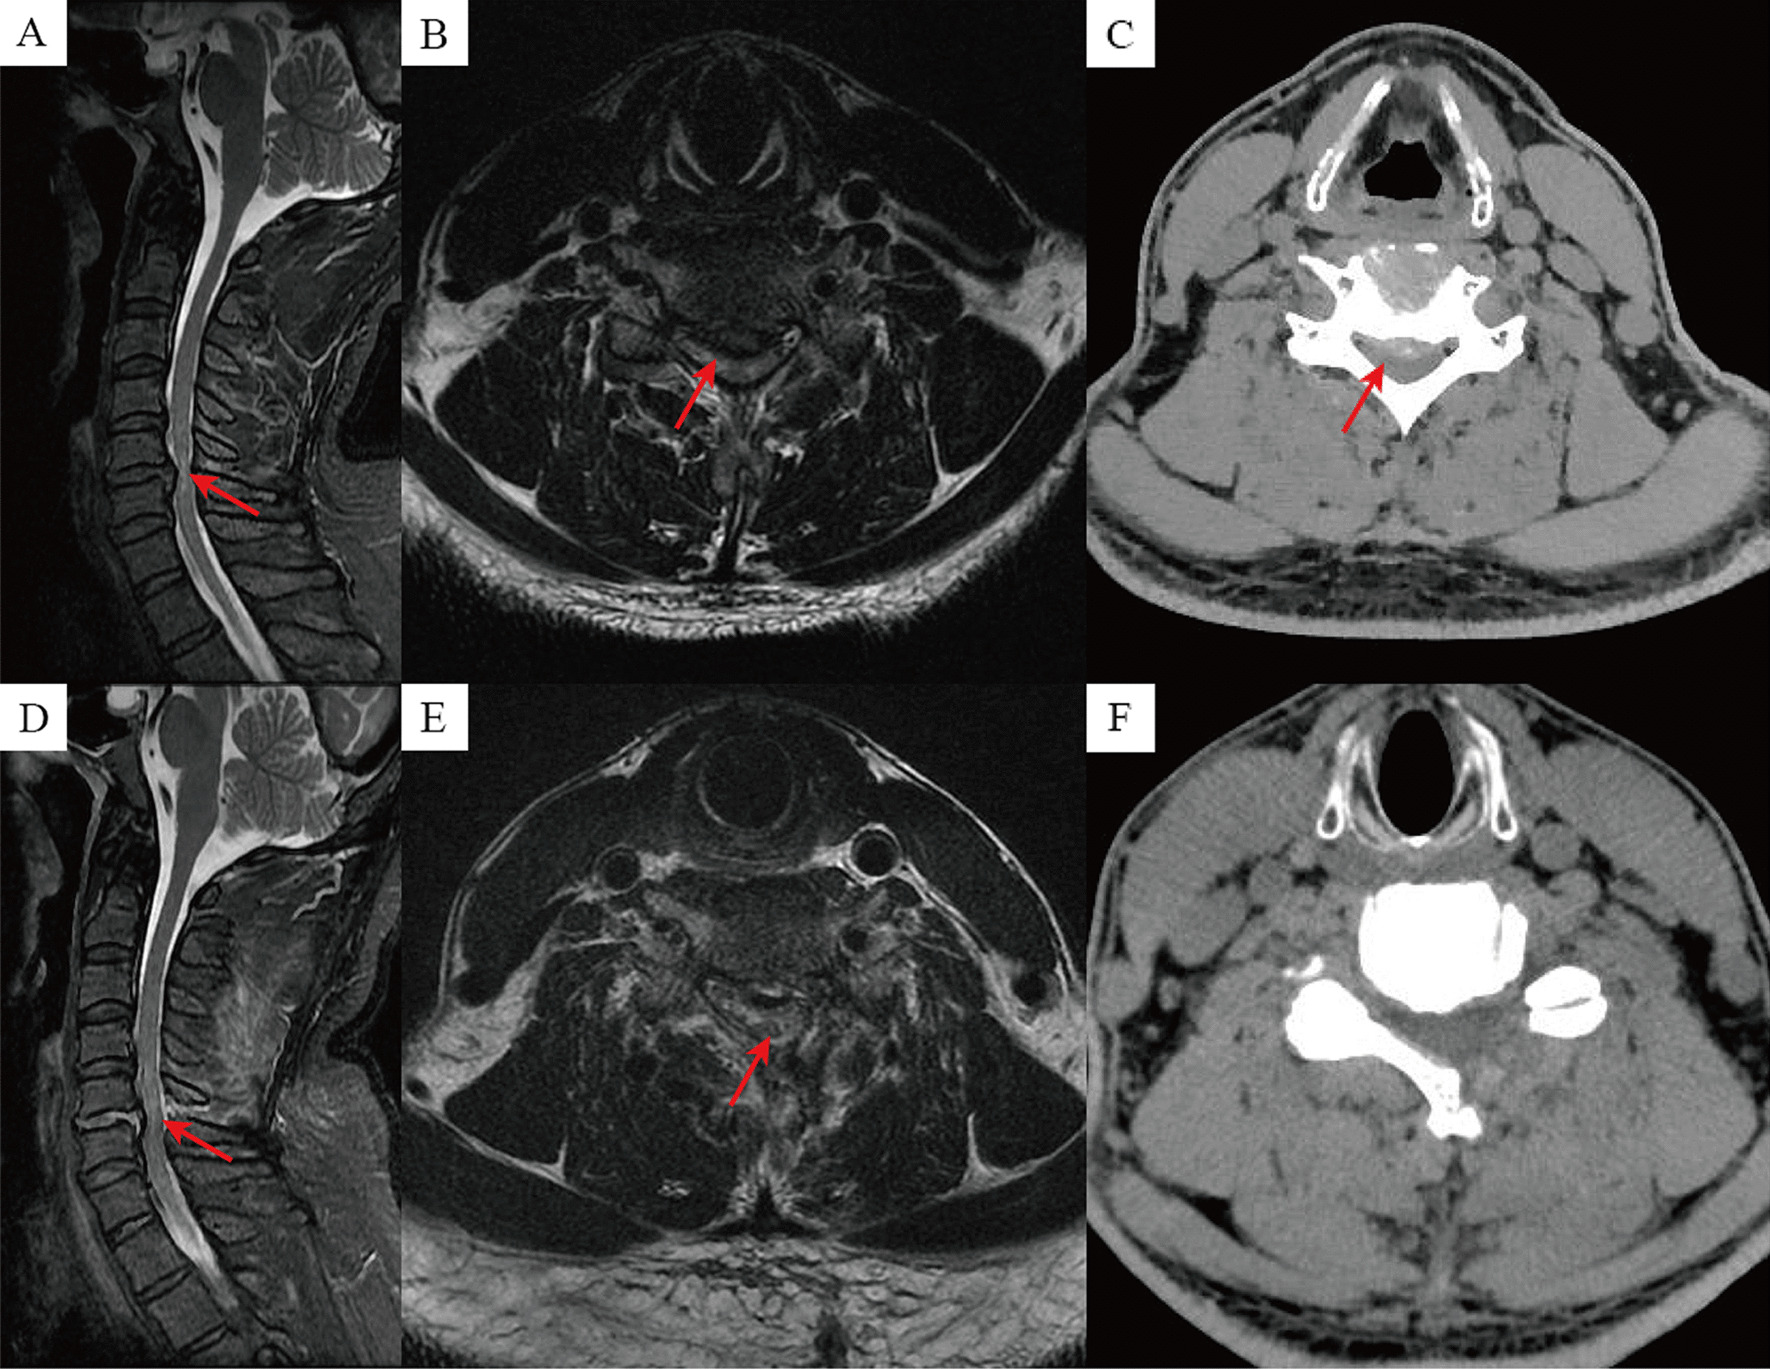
Images from a patient treated with full endoscopic laminotomy decompression. A–C Preoperative MRI and CT showed spinal stenosis of C5–6 segment, and compression of the spinal cord; D–F Postoperative MRI and CT showed adequate decompression of the spinal canal and spinal cord
